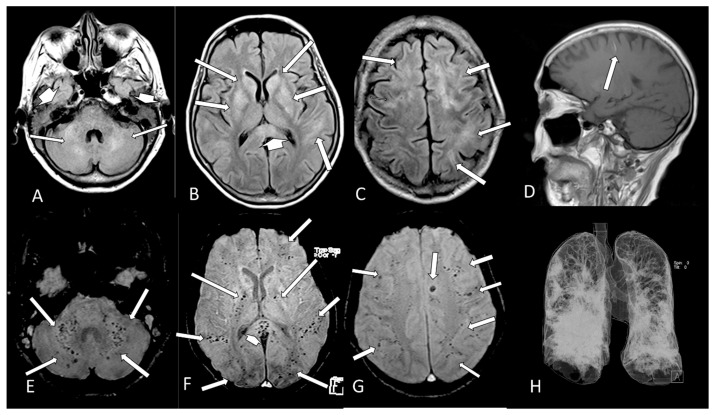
Case 30—The brain MRI shows multiple and confluent areas of diffuse hyperintensity on FLAIR (A–C, arrows) and liquid in the mastoid cells (A, short head of arrows). A small linear hyperintense subcortical on sagittal T1 (D, arrow), which represents methemoglobin, is observed. There are multiple small dots of micro bleeds at the cerebellum and middle cerebellar peduncle (E, arrows), intern capsule (F, long arrows), splenium of corpus callosum (F, head of arrow) and subcortical white matter (F,G, arrows) of the brain. The 3D chest CT reconstruction shows that there is more than 50% of opacification in both lungs (H).
